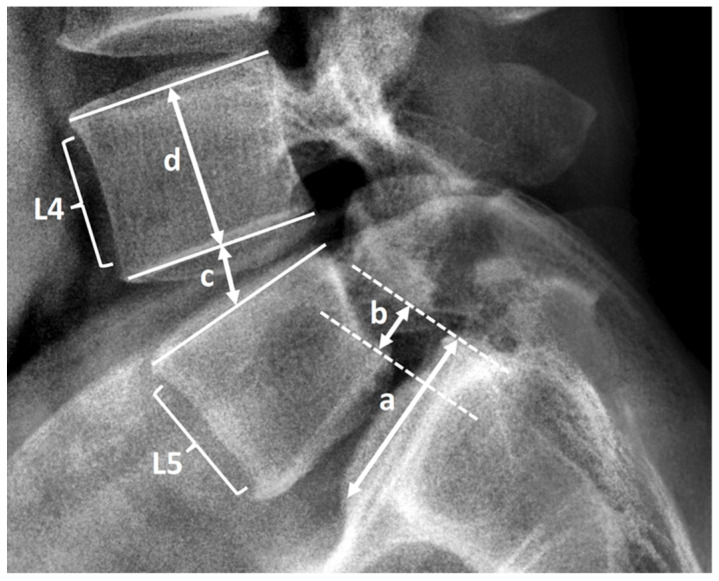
Lumbar lateral radiograph exhibits examples of L5–S1 vertebral body slippage, disc height, and vertebral body height measurement. Line segment a indicates the superior endplate of S1. Line segment b indicates vertebral body slippage length at L5–S1. Line segment c marked the distance between the midpoint of the inferior endplate of L4 and the superior endplate of L5, which indicates L4–5 disc height. Line segment d marked the distance between the midpoint of the superior endplate of L4 and the inferior endplate of L4, which indicates L4 vertebral body height.
